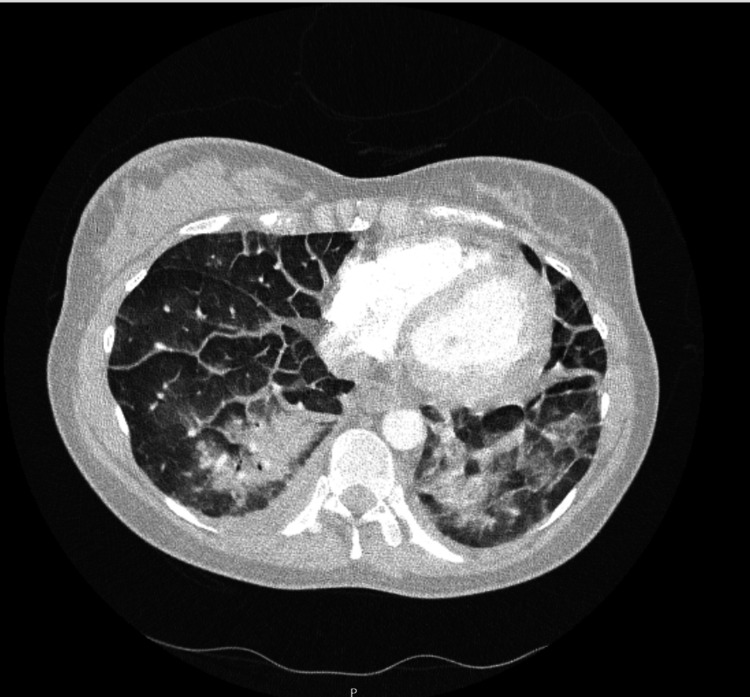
CTPA showing bilateral ground-glass opacities CTPA: computed tomography pulmonary angiogram
